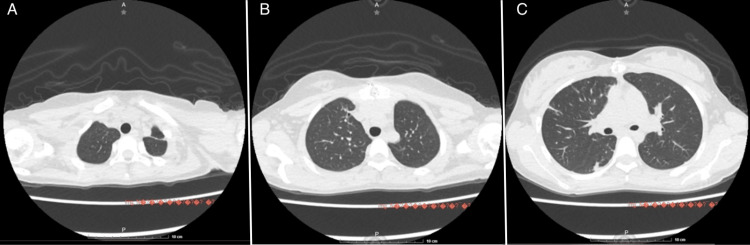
Follow-up computed tomography of the chest four weeks post-discharge A) Axial slice at the level of the lung apices showing improvement of previous lesions. B) Axial slice at the level of the middle lobe showing improvement of previous lesions. C) Improvement of the lesion in the posterior segment of the left lower lung lobe.
